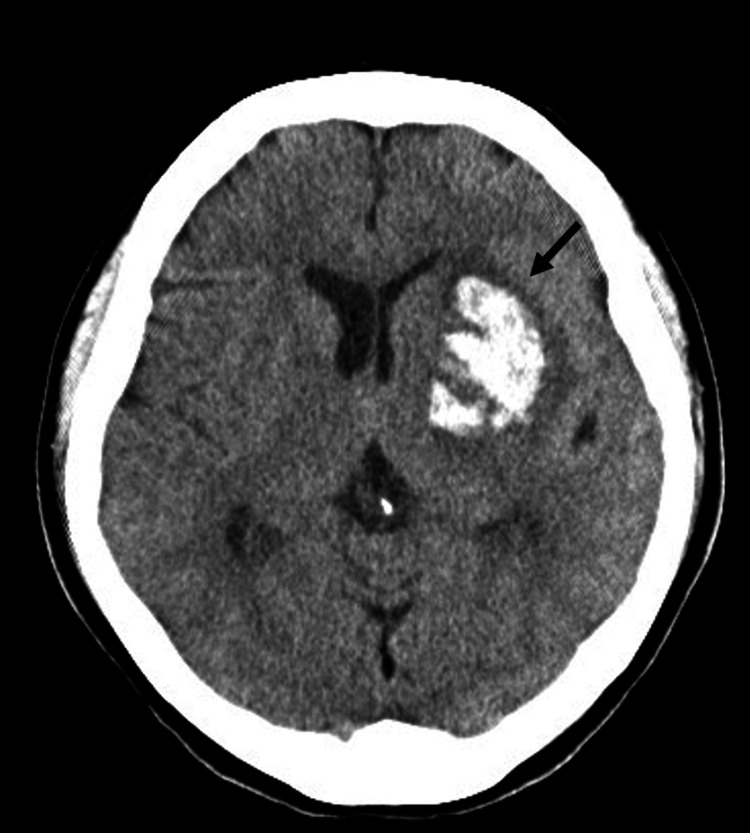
Brain computed tomography (CT) on admission. Brain CT on admission showed a hematoma in the left putamen (arrowhead). The volume of the hematoma was estimated to be 31 mL.
